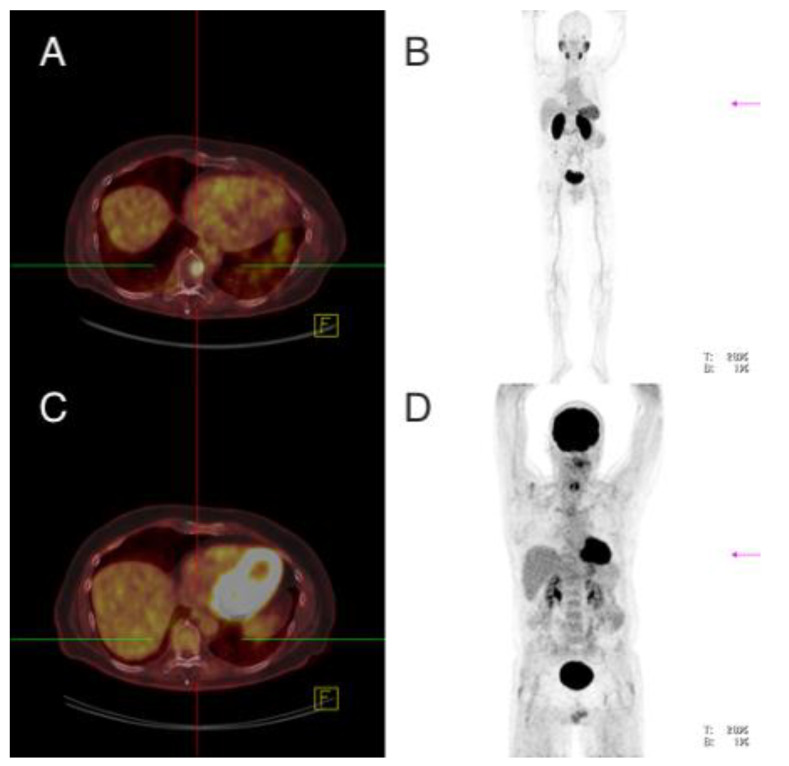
Patient 8. ^68^Ga-PSMA-PET/CT (A,B), ^18^FDG-PET/CT (C,D). Osseous metastasis thoracic vertebral body 9 with increased PSMA expression without increased glucose utilization.
